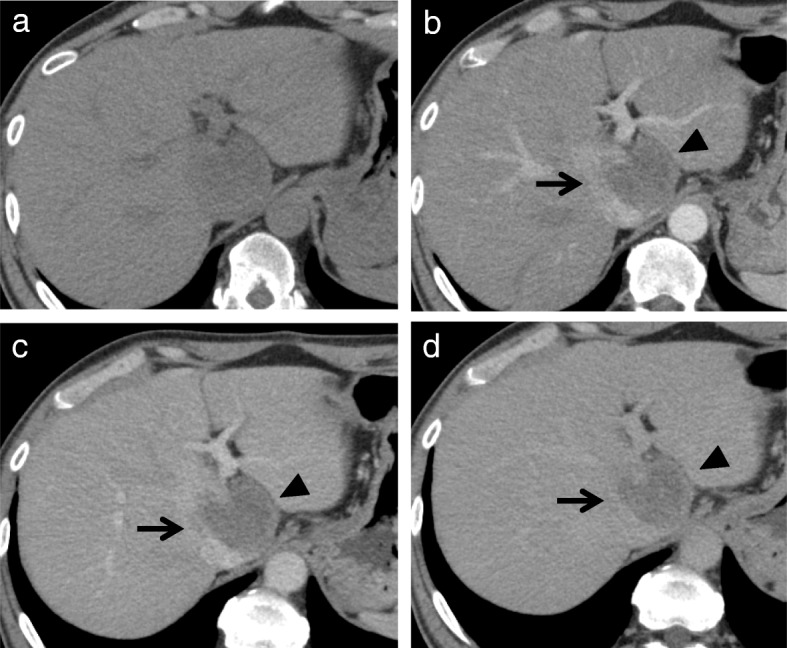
Contrast-enhanced abdominal computed tomography (CT). Plane CT scan shows a well-defined low-density mass (6.0 cm in diameter) in the caudate liver (a) Contrast-enhanced CT scan showed right component (arrow) of the tumor enhancement during the arterial phases (b) and delayed washout in the latter phases (c, d), but left component (arrowhead) as hypovascular lesion (b–d)
